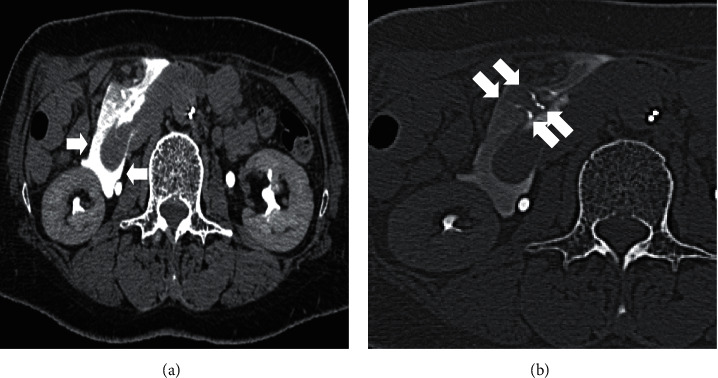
CT images of Case 1 performed immediately after embolization. CT showed leakage of the contrast medium into the retroperitoneum ((a), arrows). Lipiodol retention was observed in the duodenal arteries ((b), arrows). No free air was observed in the abdomen.
